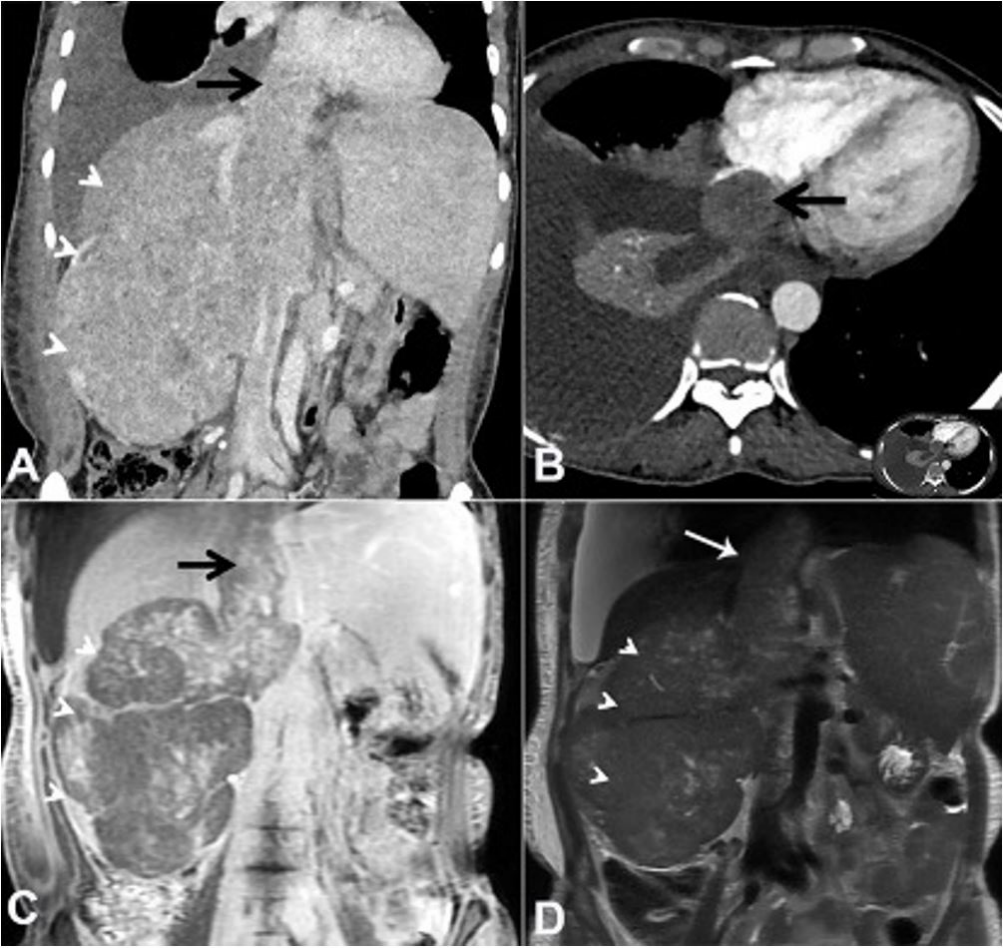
A – contrast-enhanced abdominal CT, Coronal plane, showing heterogeneously enhancing mass lesion (arrowheads) almost replacing the right kidney with tumor thrombus (arrow) extending into the right renal vein and IVC; B – axial plane showing a tumor mass within the right atrial chamber (arrow) (inset represents the whole slice); C – Coronal T1-weighted post-contrast abdominal MRI showing patchy moderate central enhancement (arrowheads) with enhancement of thrombus (arrow) expanding the IVC; D – Coronal T2-weighted abdominal MRI showing relatively isointense mass lesion (arrowheads) with interspersed hyperintense areas consistent with necrosis.
